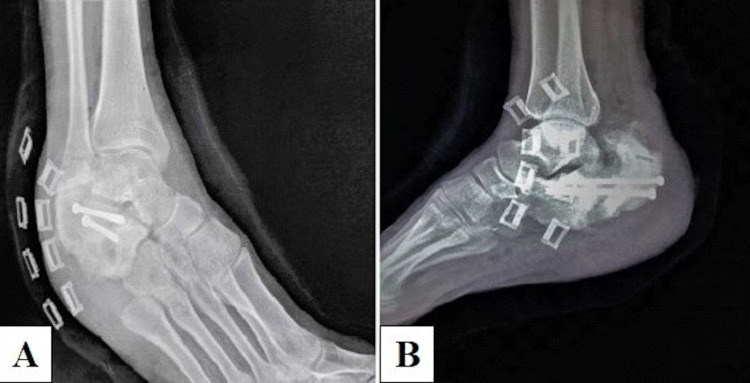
Immediate postoperative radiographs showing acceptable reduction and adequate compression. (A) Oblique view. (B) Lateral view.
